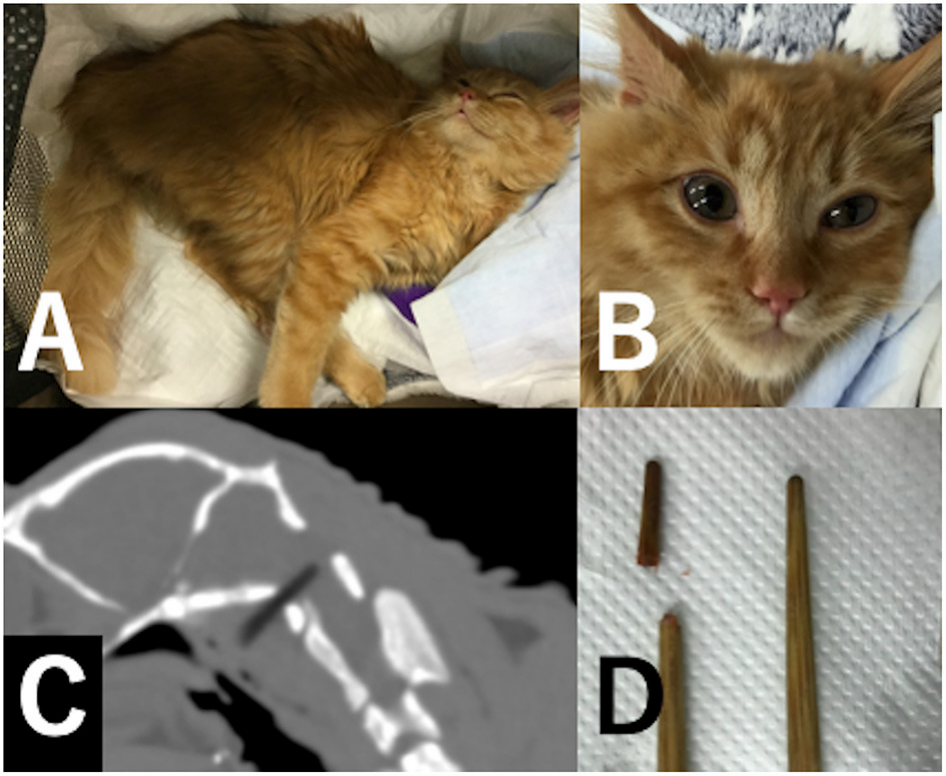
Pictures and diagnostic images in case 2. At presentation, a decreased level of consciousness, tetraparesis (A), and Horner's syndrome (B) were observed. A low-attenuation linear structure from the oral cavity passing through the atlantooccipital space was found on CT examination (C). The fragment of the chopstick was removed surgically (D).
